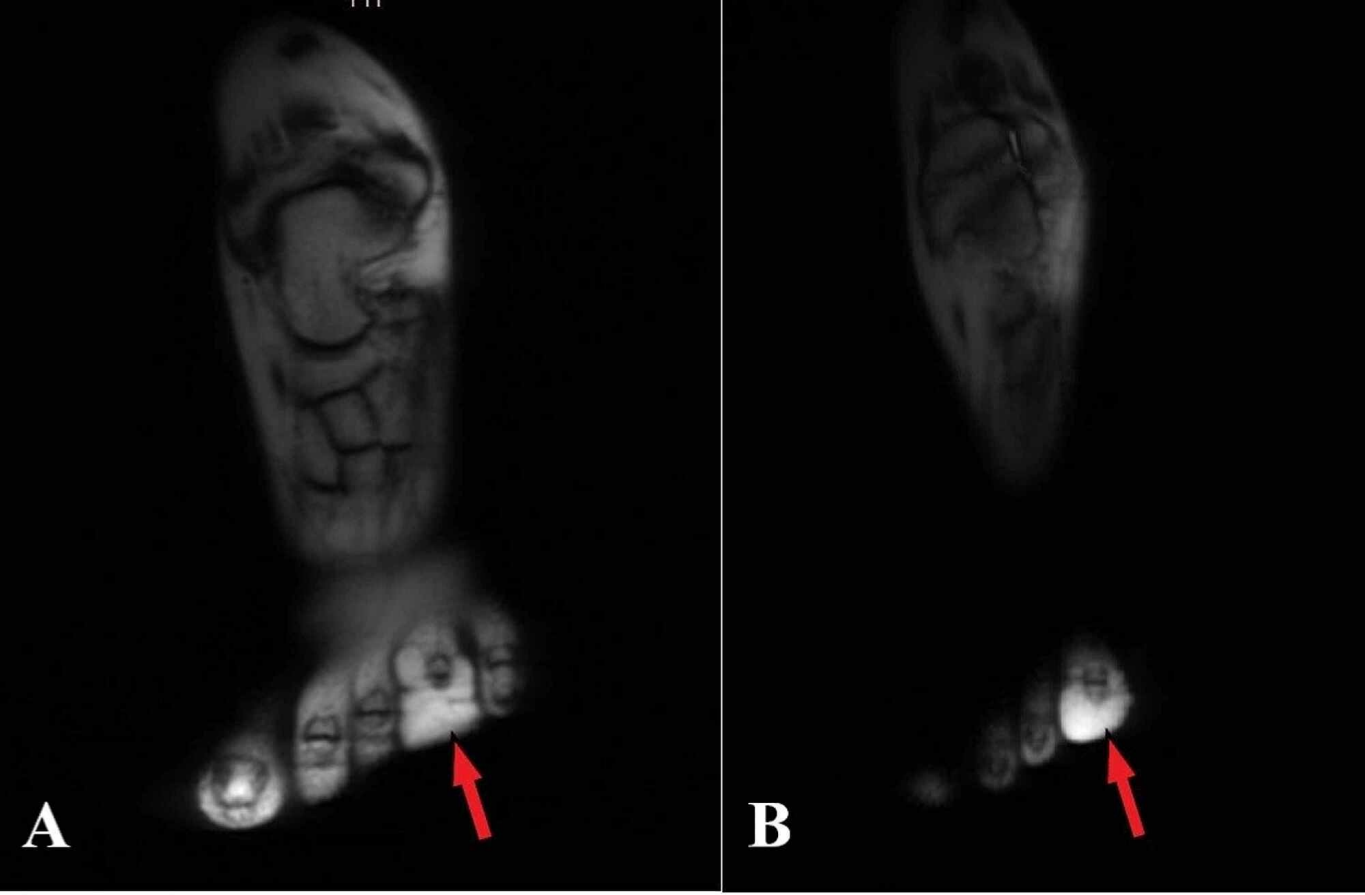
MRI images of toe macrodactyly. MRI shows lobulated large subcutaneous homogeneous soft tissue mass of fat signal intensity with internal fibrous strands located around the left fourth toe (red arrow in A and B).
